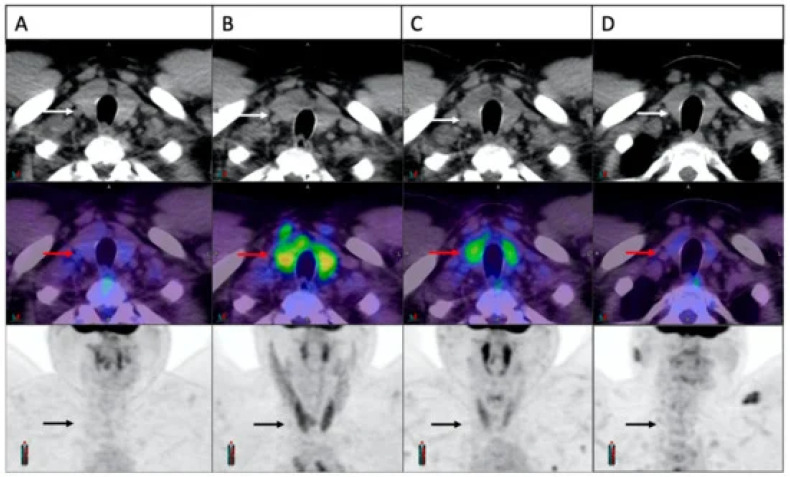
Four FDG-PET scans showing a patient with ICI-induced thyroiditis and the evolution of thyroid FDG uptake over time. Initially, the thyroid appeared normal ((A), arrows). Three weeks into treatment, there was a notable rise in FDG uptake ((B), arrows), followed by partial resolution at 6 weeks ((C), arrows) and complete resolution at 24 weeks ((D), arrows). The material was originally published in Galligan et al. (2023) [56].
